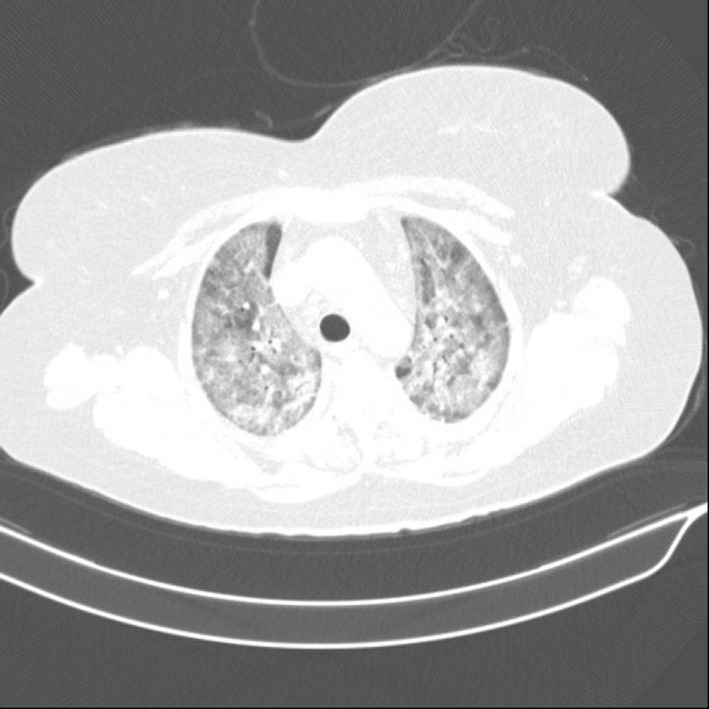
Chest computed tomography (CT) scan revealed diffuse ground‐glass opacity in both lungs with crazy‐paving in favor of COVID‐19 pneumonia
